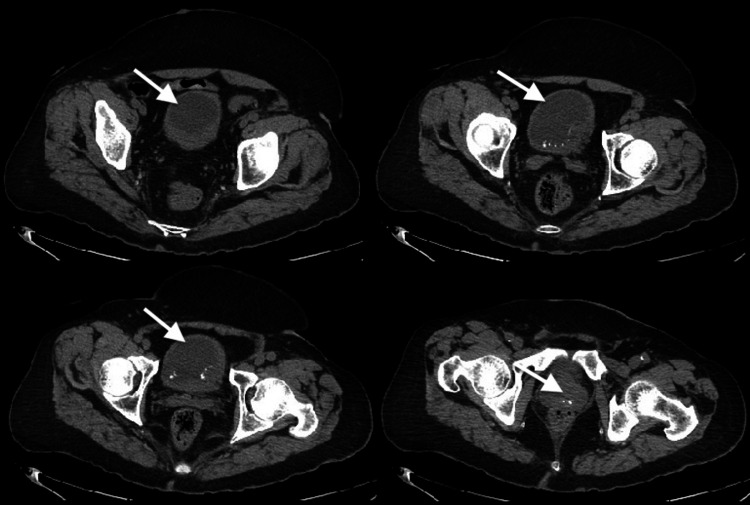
CT pelvis at various levels (white arrows) showing heterogenous hyperdense mass of the bladder suggestive of a bladder tumor with several calcifications.
